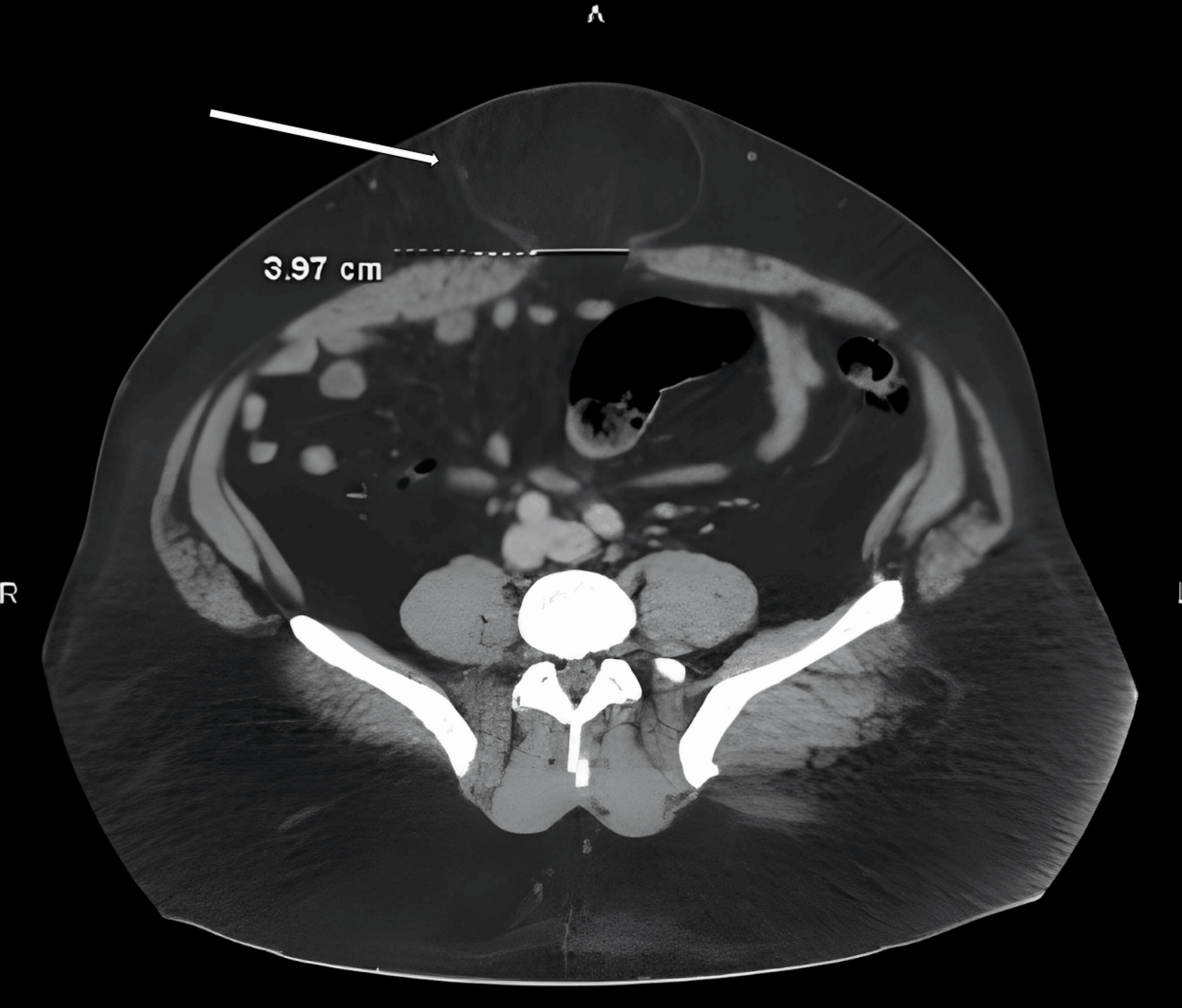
Transverse CT scan before hernia surgery showing hernia defect with the protruding hernia sac (white arrow) CT: computed tomography
